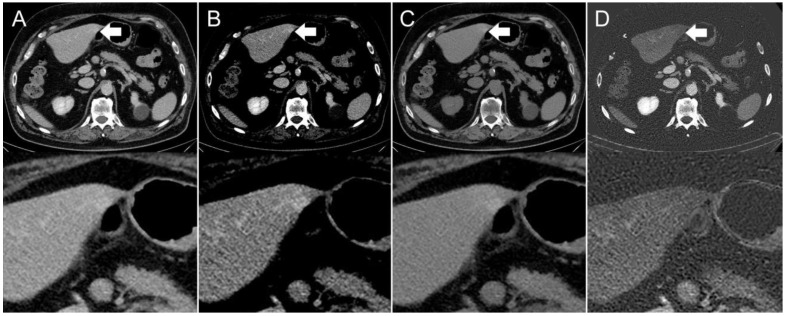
Axial contrast-enhanced CT scan of a 67-year-old man. Hyperdensity (indicated by arrows) on the left liver lobe in the (A,B) conventional 120 kVp image using an (A) abdomen window and (B) liver window, which might be interpreted as a hyperenhancing liver lesion. The (C) virtual non-contrast (VNC) image reconstruction and (D) iodine image reconstruction reveal that it is not a hyperenhancing liver lesion, but a streak artifact originating from the stomach antrum.
